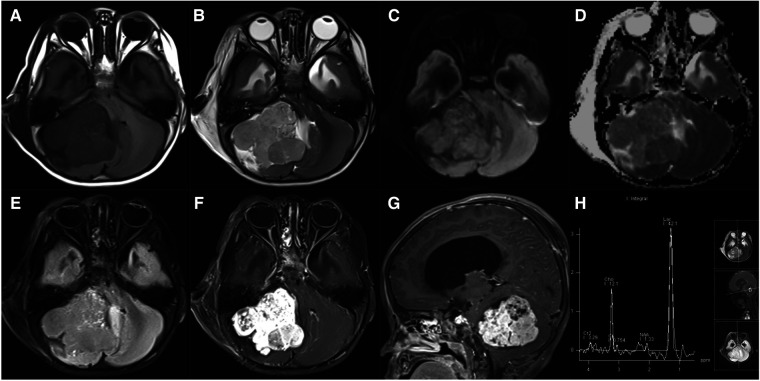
22-month-old boy with a cerebellar yolk sac tumor: axial T1WI (A), T2WI (B), DWI (C), ADC maps (D), FLAIR (E), and post-contrast T1WI (F), sagittal post-contrast T1WI (G), and MRS (H) show an irregular, heterogeneous mass with marked enhancement, diffusion restriction, and decreased NAA peak.
